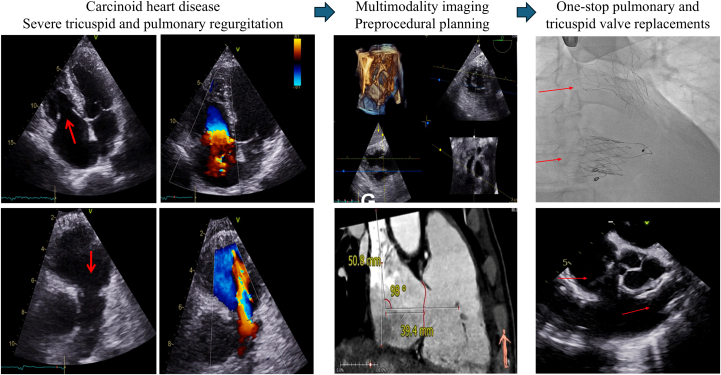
Right-Sided Carcinoid Heart Disease Diagnosis, Preprocedural Imaging and Planning, and One-Stop Transcatheter Valve Replacement Results Through Fluoroscopy and Echocardiography
